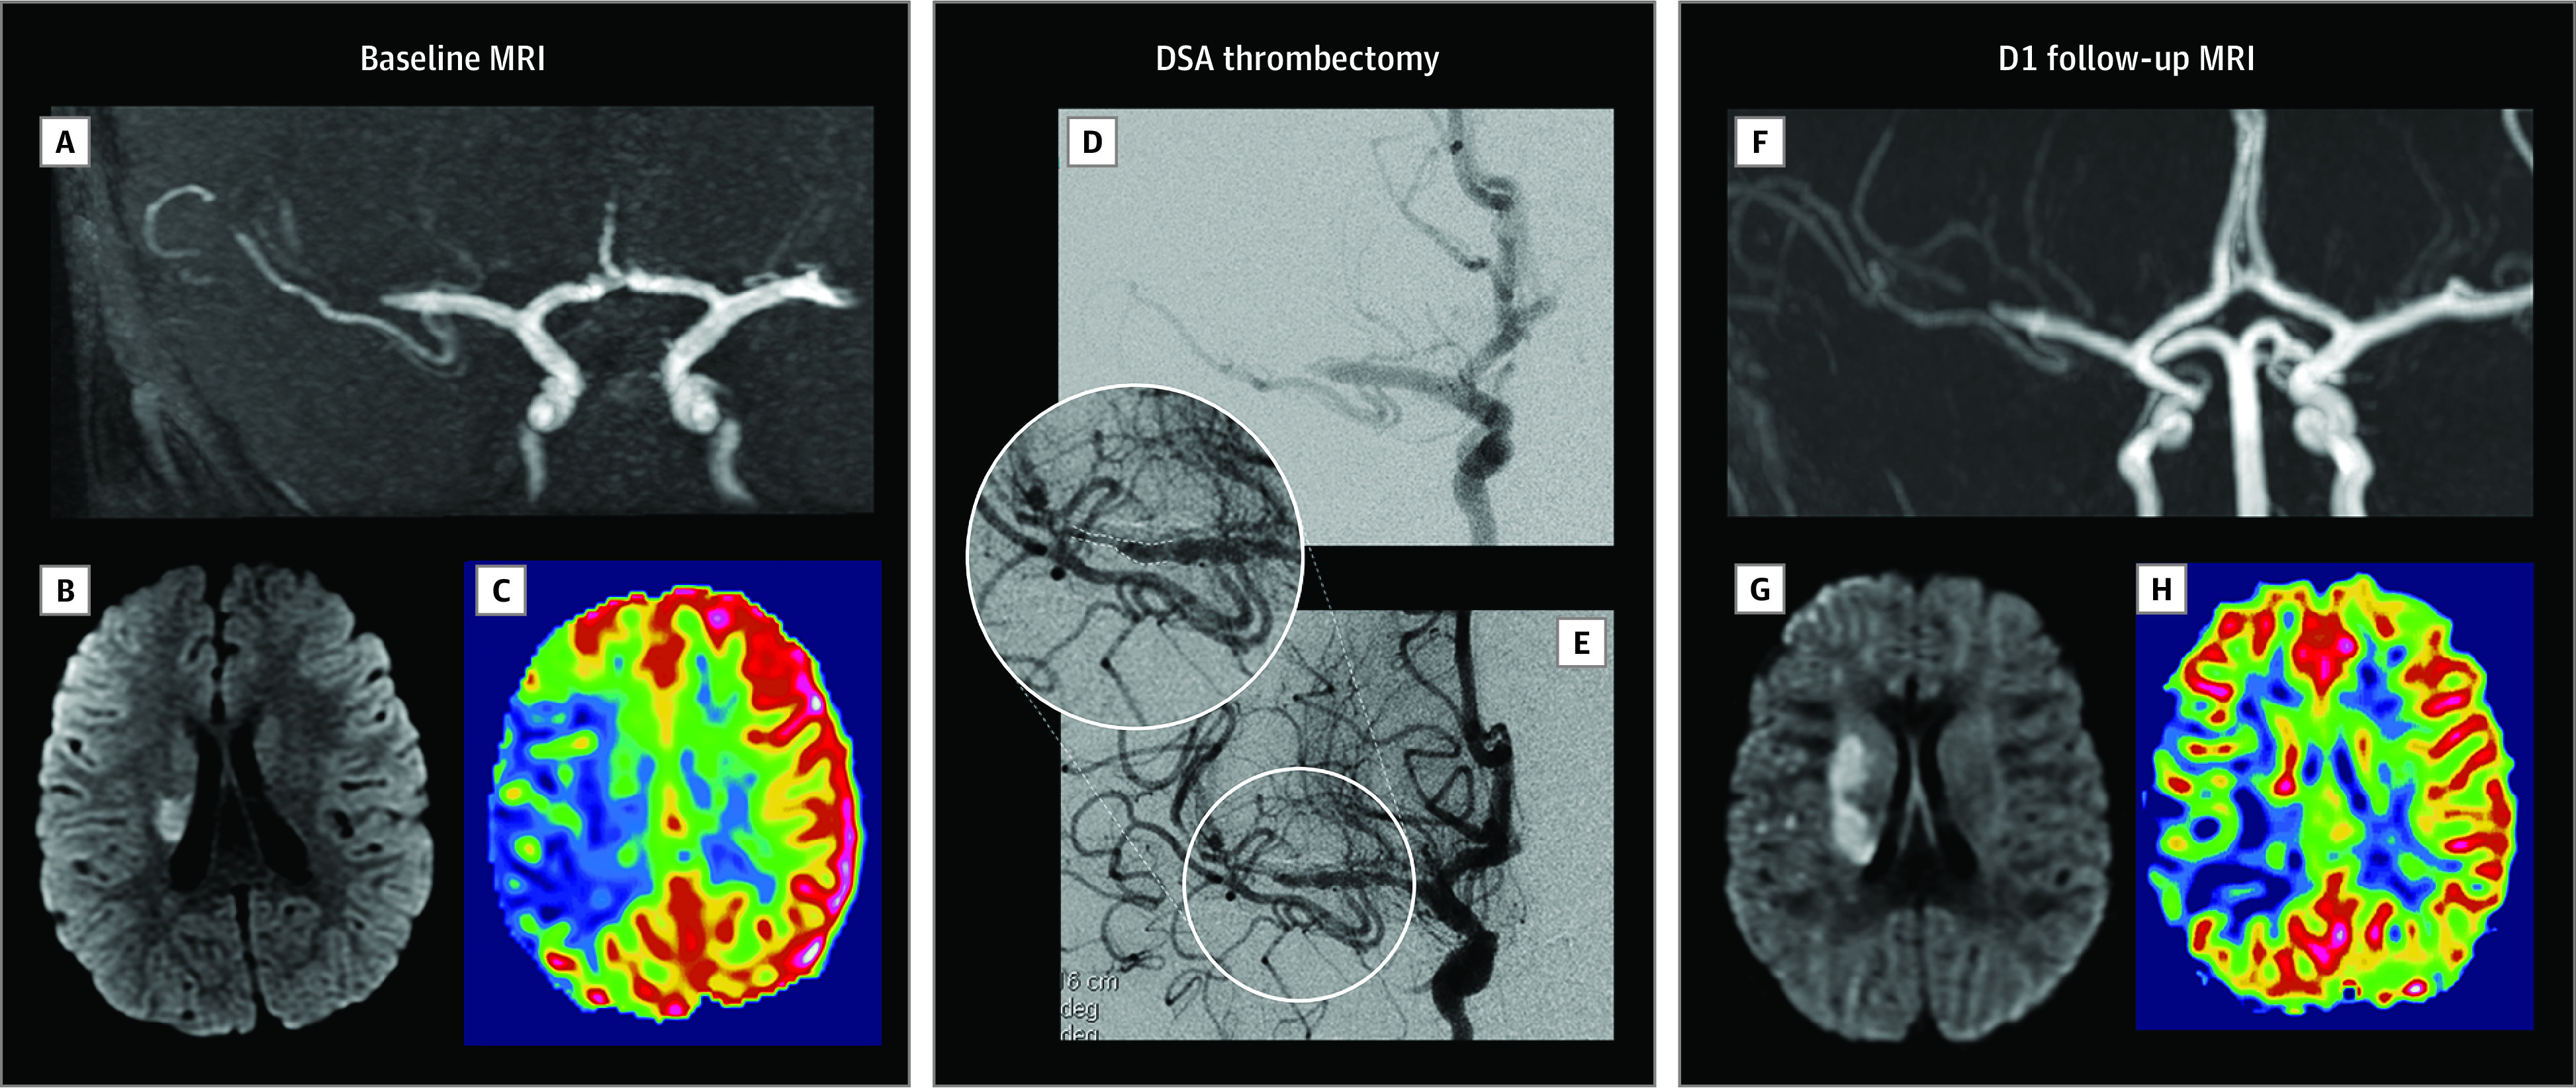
Pediatric Acute Ischemic Stroke Caused by FCA Treated With Mechanical Thrombectomy Acute ischemic stroke in a child aged 4 years with no medical history, presenting as a sudden left hemiplegia and facial palsy. A-C: Baseline magnetic resonance imaging (MRI) performed 2 hours and 50 minutes after stroke onset. A, Coronal maximum intensity projection reconstruction of time-of-flight angiography showing the occlusion of the distal M1 segment of the right middle cerebral artery (MCA). B, Axial diffusion image showing hyperintensity limited to right caudate. C, axial arterial spin labeling with cerebral blood flow (ASL-CBF) cartography showing markedly decreased perfusion throughout the right sylvian territory compared with the left side. D-E: Periprocedural digital subtraction angiography (DSA) images. D, Initial anteroposterior images after opacification of the right carotid territory confirming the occlusion (distal M1 segment) of the right MCA. E, Final anteroposterior images after mechanical thrombectomy with stent retriever showing restored patency of the right MCA; however, with significant residual stenosis. F-H: Follow-up MRI performed 24 hours (D1) after the mechanical thrombectomy. F, Axial MIP reconstruction of time-of-flight images showing the reclusion of the stenosed MCA. G, Axial diffusion image showing the extension of the ischemic core to the caudate nucleus and the posterior limb of the internal capsule; note the small focal ischemic spots in the superficial MCA territory. H, Axial ASL-CBF cartography showing the remaining decreased perfusion in the right MCA territory.
